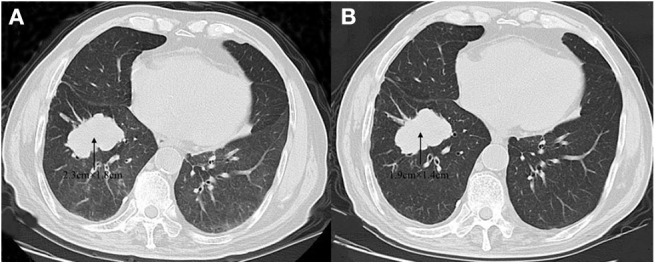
Computed tomography (CT) scans before (A) and after (B) afatinib therapy.
